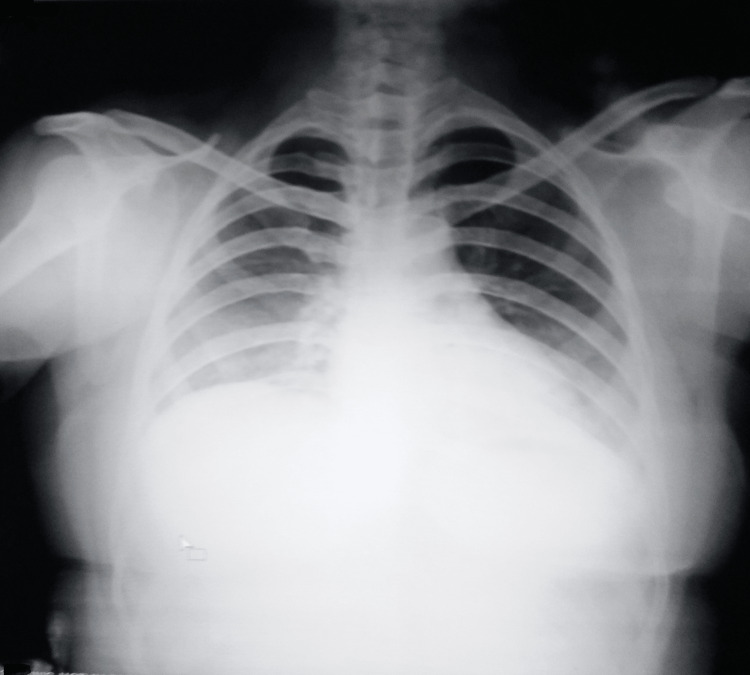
An unremarkable chest radiograph (PA view) PA: posteroanterior
